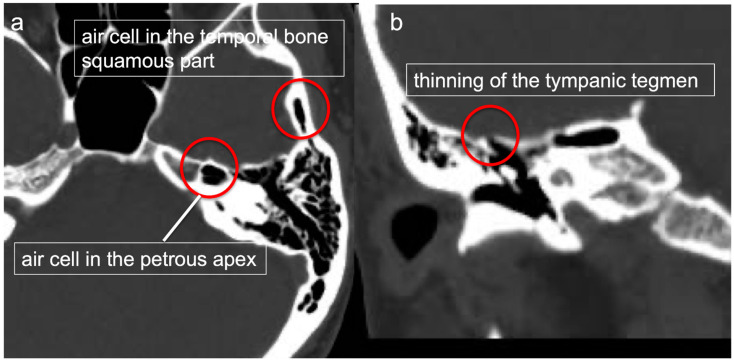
Preoperative thin-slice CT. The presence of air cells in the temporal bone squamous part and petrous apex (a) and thinning of the tympanic tegmen (b) were confirmed via thin-slice CT.
